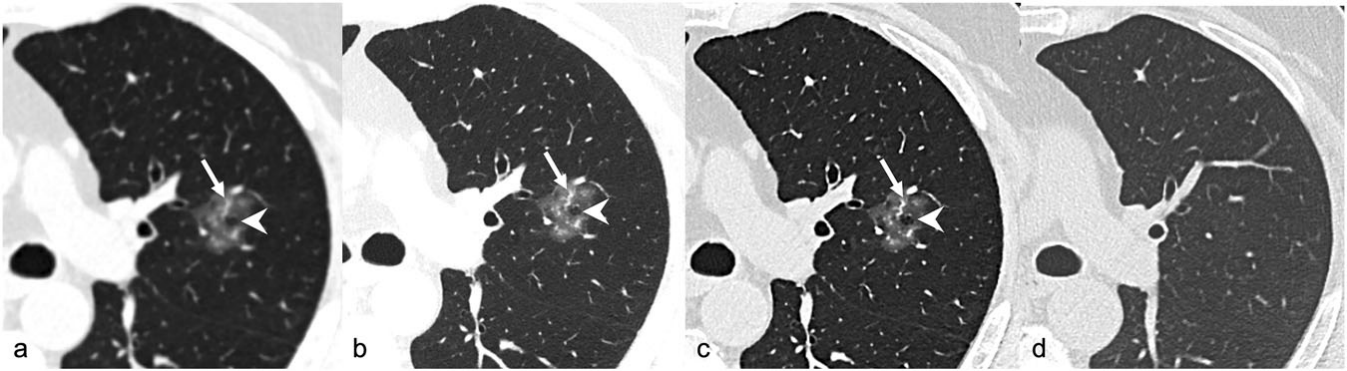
Axial chest PCCT scan of a patient with a pure ground-glass lung nodule in the upper left lobe. The inflammatory nodule shows ill-defined margins, bubble-like lucencies (arrowhead) and a serpiginous dilated vessel (arrow) within it. The characterization of these peculiarities of the nodule improves as matrix size increases (from 512 × 512 (a) to 1,024 × 1,024 (b, c), slice thickness decreases (from 1 mm with 0.6 slice increment in a and b to 0.4 mm with 0.2 slice thickness in c) and a dedicated reconstruction kernel for lung (Bl60) is used (c). The inflammatory ground-glass nodule, along with the inner vessel dilation and bubble-like lucencies, completely disappeared at follow-up scan (d), acquired by a conventional EID-CT scanner (1.25 mm slice thickness, 0.6 mm increment, matrix size 512 × 512, Br56 reconstruction kernel)
